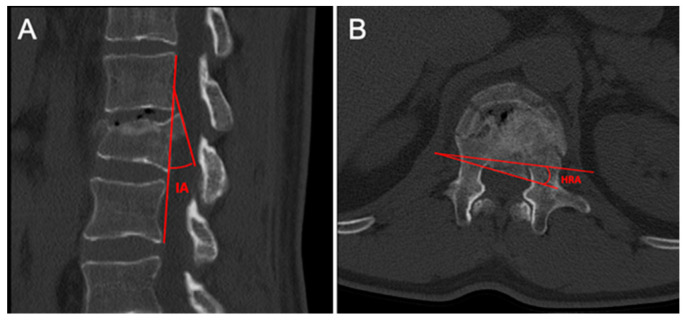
CT bone fragment parameters. (A) IA (inversion angle). (B) HRA (horizontal rotation angle).
